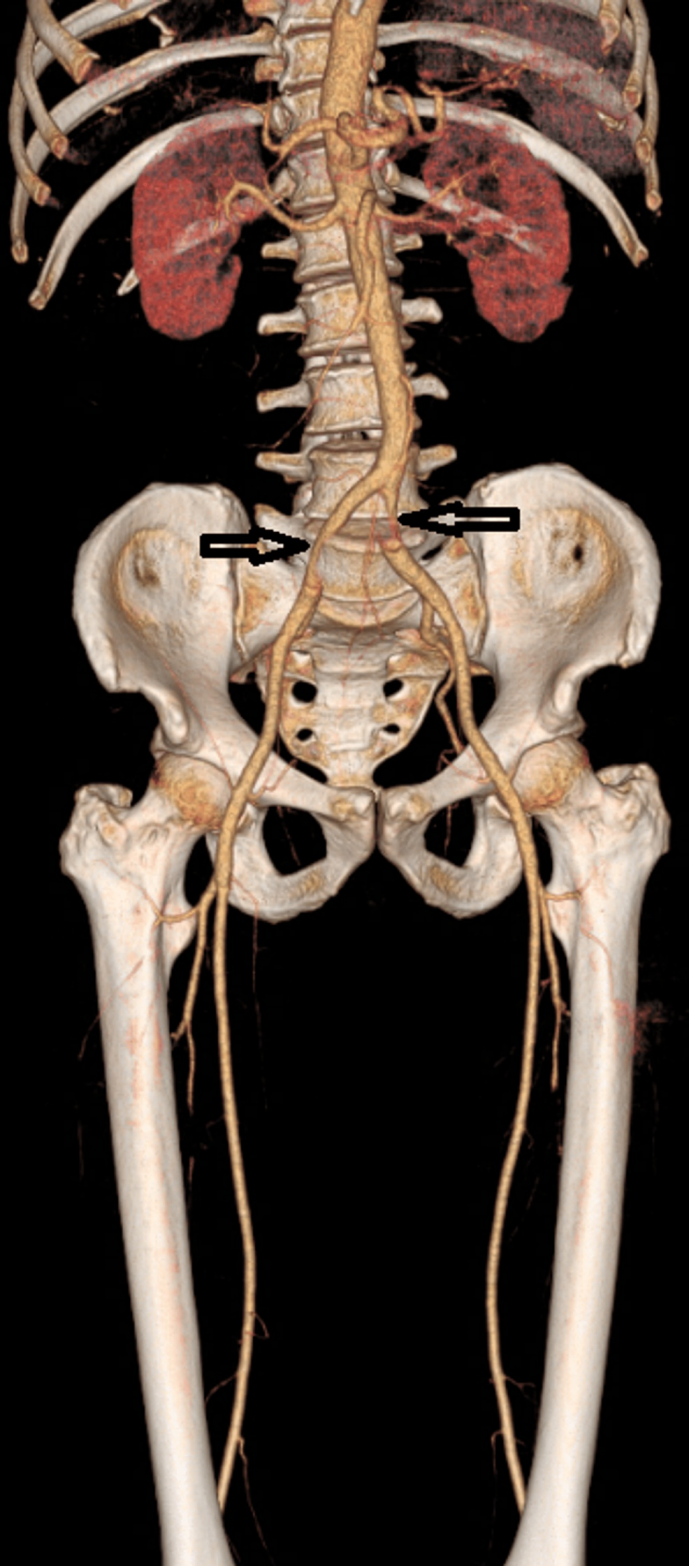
CT angiography on 3D reconstruction showing a bilateral common iliac artery partial thrombosis (black arrows)
